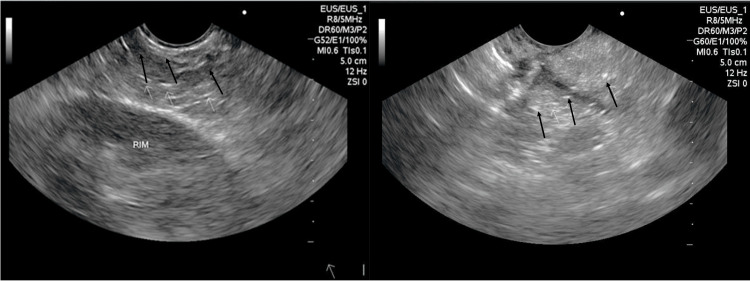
Endoscopic ultrasound Diffuse hyperechoic strands in the parenchyma (arrows) suggestive of initial stage of chronic pancreatitis.
